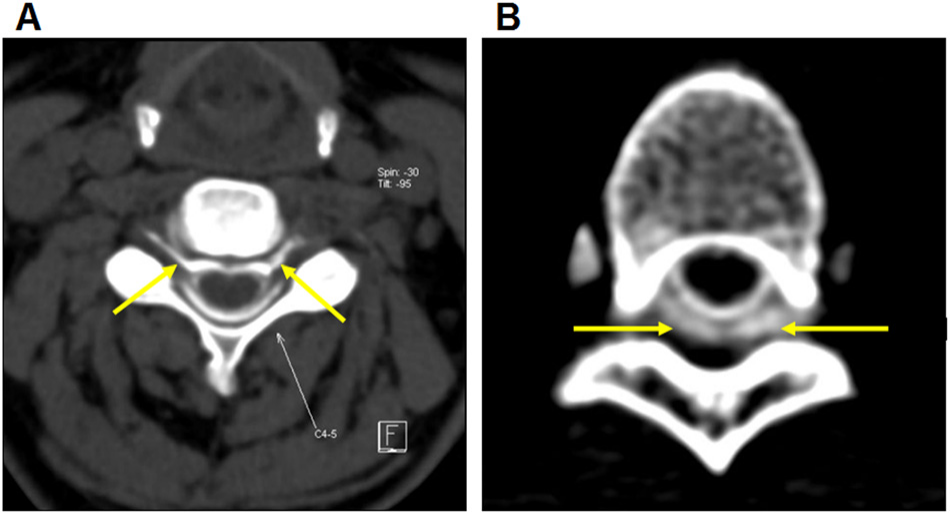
CT myelography for evaluating dural leakage. CT myelography shows contrast tracking along bilateral nerve roots (A, arrows) and collections of contrast within the paraspinal soft tissue (B, arrows). Images taken at segments C4-5 (A) and T5-6 (B).
